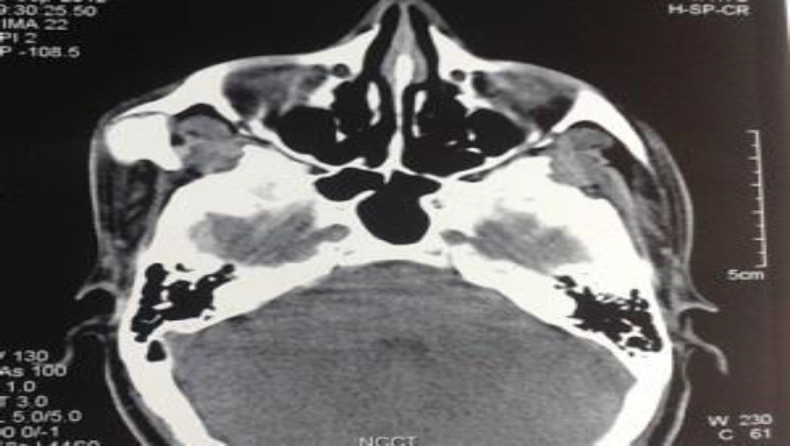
Axial Computed tomographic image revealing elongated coronoid causing thinning and bulging of zygoma
